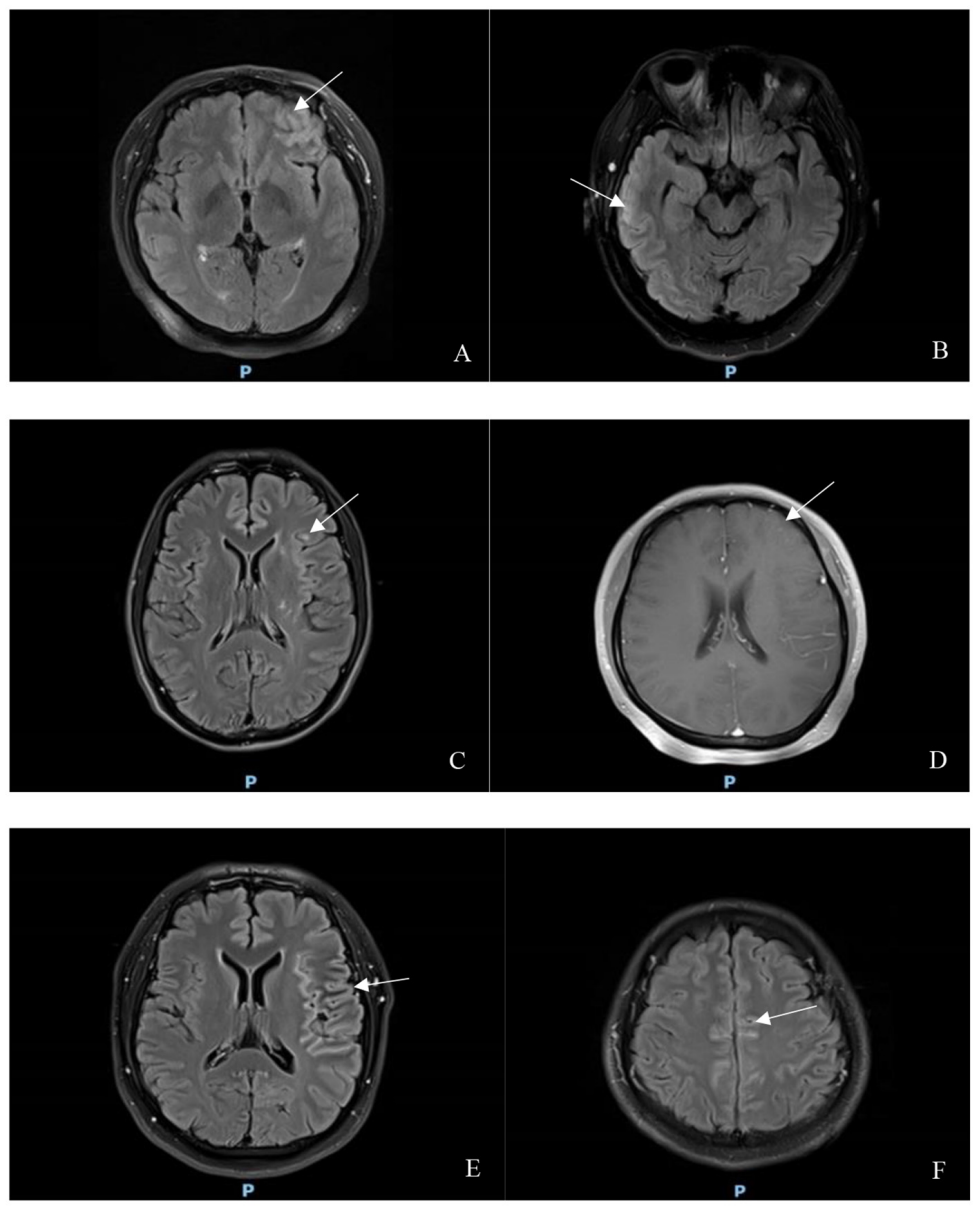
(A) shows a high FLAIR signal in the left forehead; (B) shows a high FLAIR signal in the right temporal lobe; (C) shows a high FLAIR signal in the left temporal and insular lobes; (D) shows left frontal leptomeningeal enhancement on the contrast-enhanced T1-weighted sequence; (E) shows FLAIR enhancement changes in the left temporal leptomeninges; (F) shows FLAIR enhancement changes in the leptomeninges adjacent to the midline of both frontal and parietal regions.
